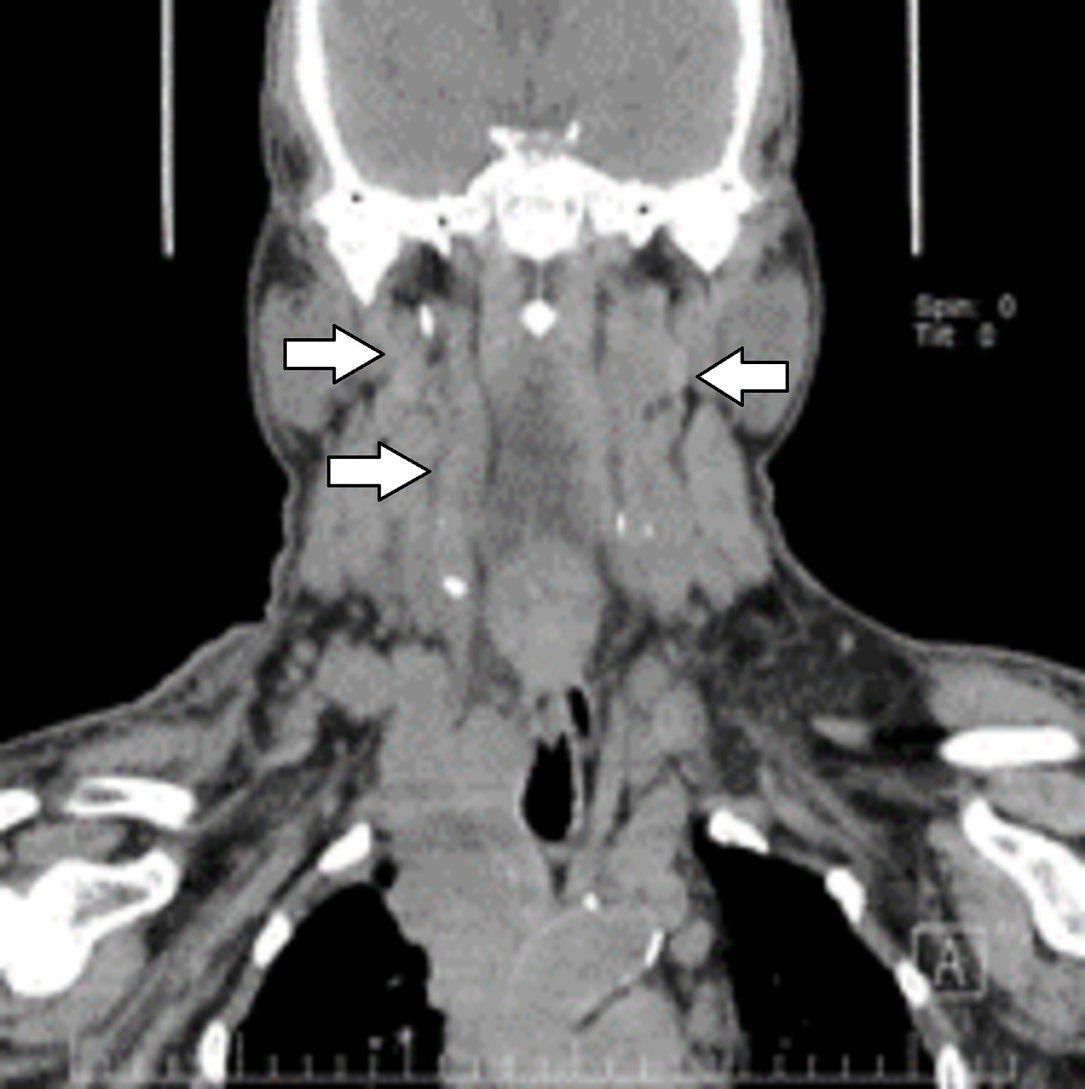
Coronal head and neck computed tomography. Bilateral adenopathy; in the right neck at levels IIA, IV (right arrows); left neck at level III (left arrow).
